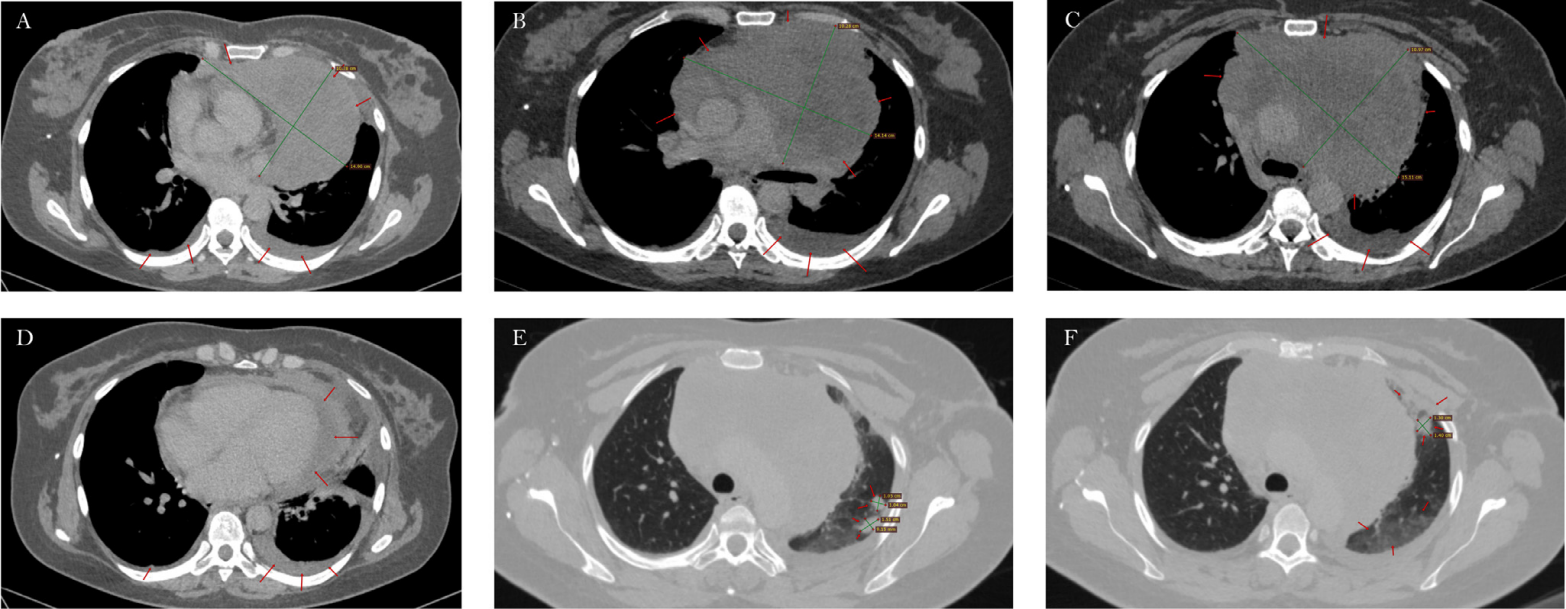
Follow-up CT scan after 3 months. Visible changes in tumor size and metastatic disease – axial view (A, B, C). Pericardial and left-sided pleural effusion.
